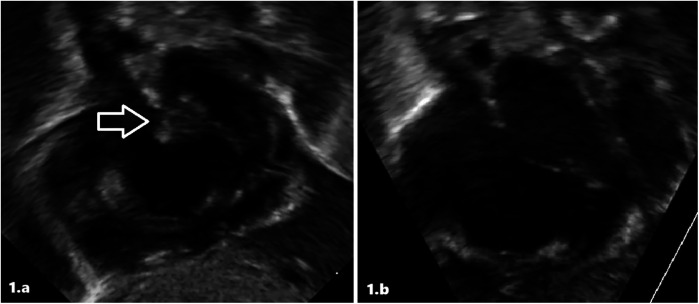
Intracardiac thrombus at diagnosis (a) and after treatment (b) in patient 1 at echocardiographic scans.
